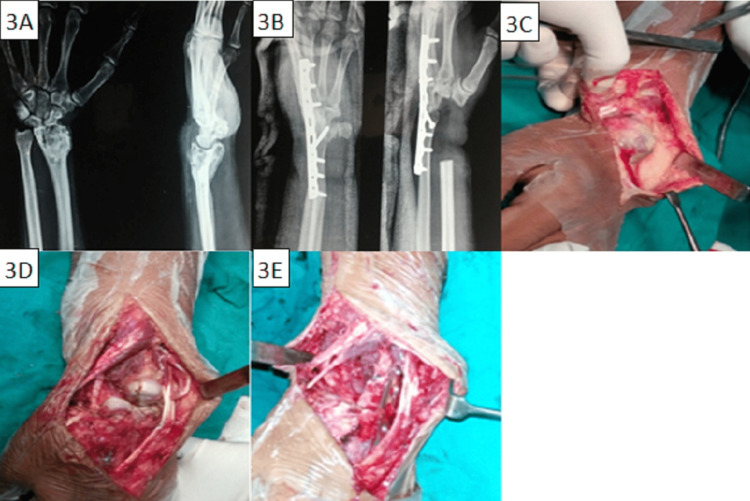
Recurrent GCT of distal end radius in a 20-year-old female. (A) Preoperative radiographs showing recurrent GCT of distal end radius; (B) Postoperative radiograph showing resection and wrist arthrodesis with centralization of ulna; (C), (D), (E) Intra-operative pictures GCT: giant cell tumour
